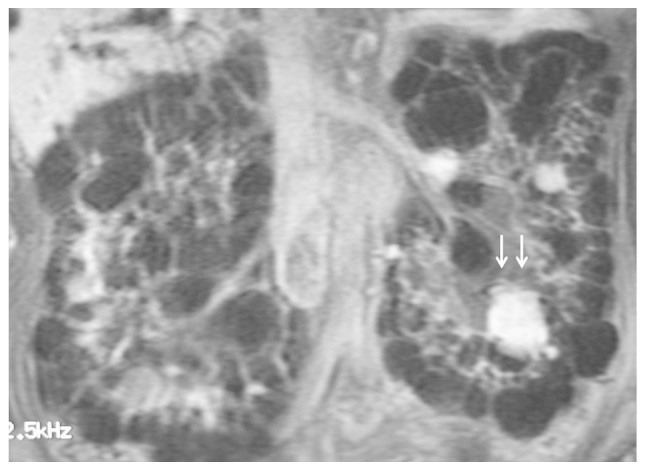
Magnetic resonance imaging (coronal view) revealing a highly enhanced tumor (white arrows) in the lower part of the left polycystic kidney.
